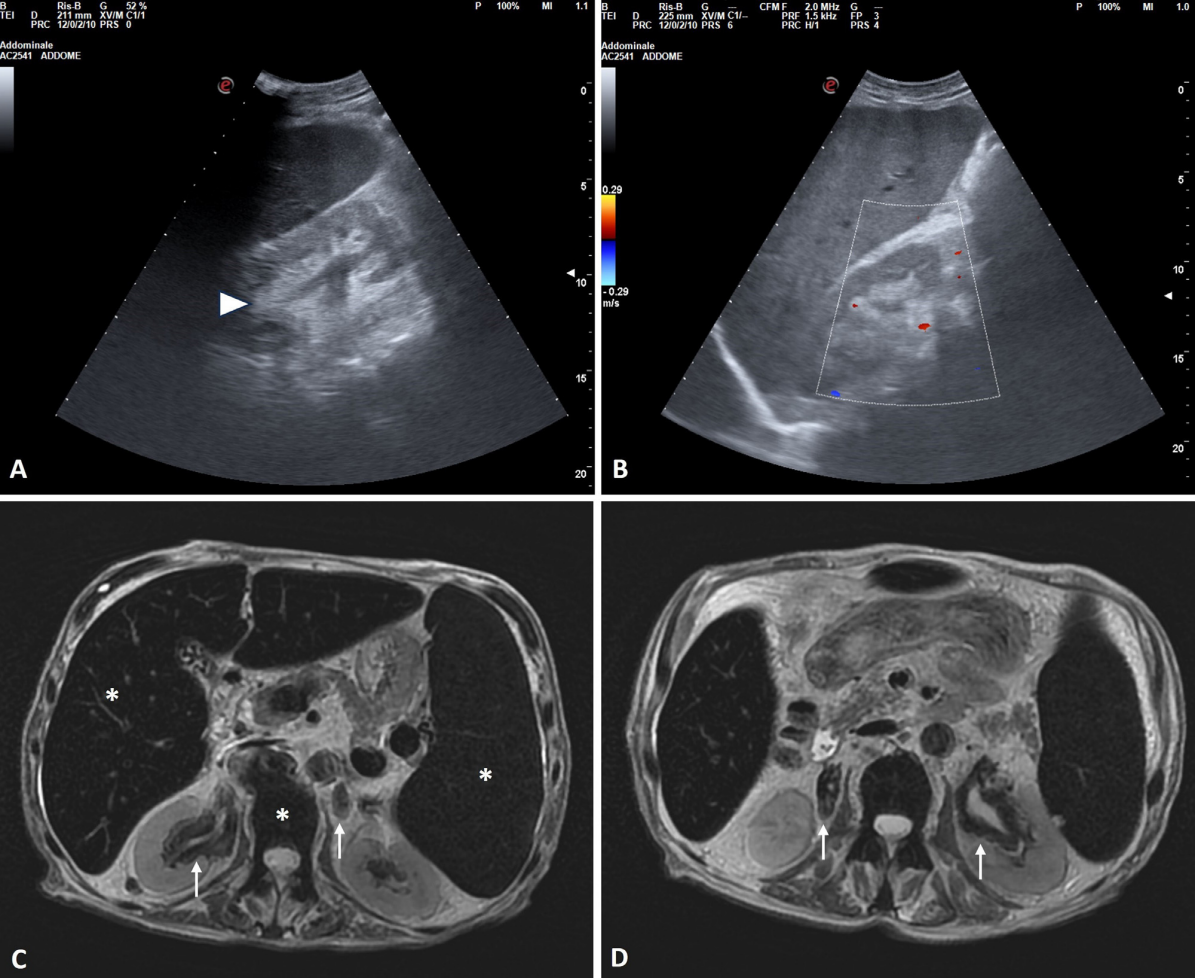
Imaging features of renal extramedullary hematopoiesis. (A, B) On ultrasound examination the right kidney shows hyperechoic tissue around the hilar and pelvic region (white arrowhead) extending to the proximal portion of ureter (A), with vessels visualization on color-doppler examination (B). (C, D) Axial T2-weighted turbo spin-echo images show parapyelic, peri-hilar and peri-ureteral solid bilateral renal lesions with infiltrative pattern and low signal intensity (white arrows). Signs of iron overload due to secondary hemochromatosis are evident in the liver, spleen and bone marrow with marked low signal intensity on T2-weighted turbo spin-echo sequences (white asterisks). Note splenomegaly.
